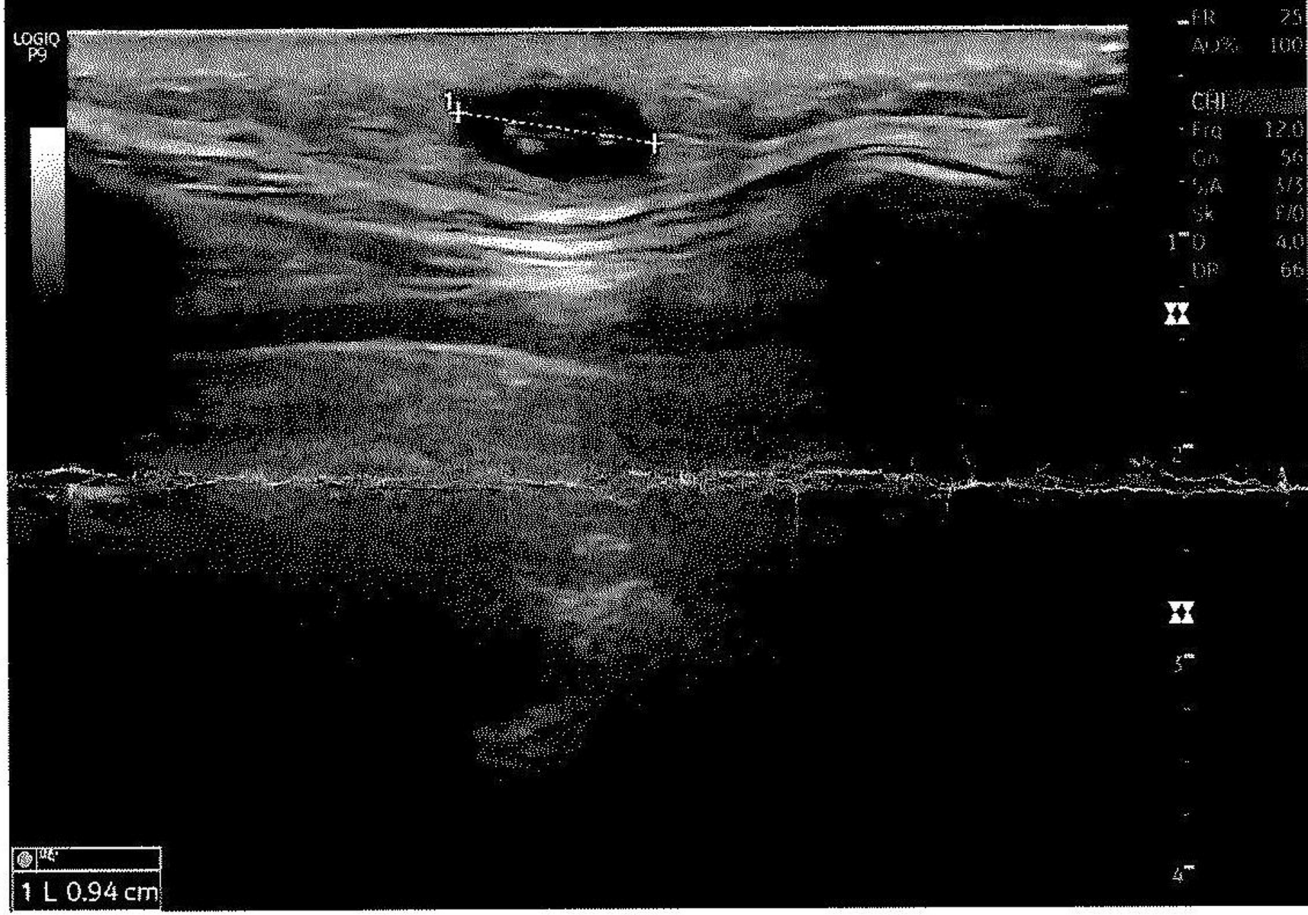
Ultrasound image showing a subcutaneous, hypoechoic, oval, 0.9 cm nodule with a central linear mass of 0.5 cm
